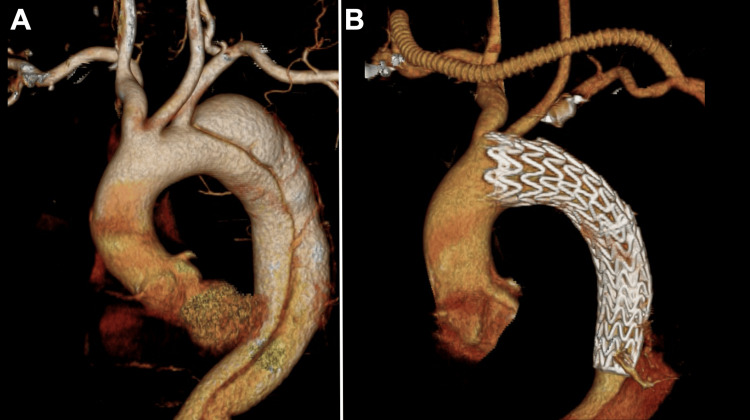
Thoracic endovascular aortic repair for type B aortic dissection. Computed tomography angiography shows a type B aortic dissection and a thoracic descending aortic dissecting aneurysm (A). Thoracic endovascular aortic repair with a right-to-left axillary artery bypass was performed (B).
